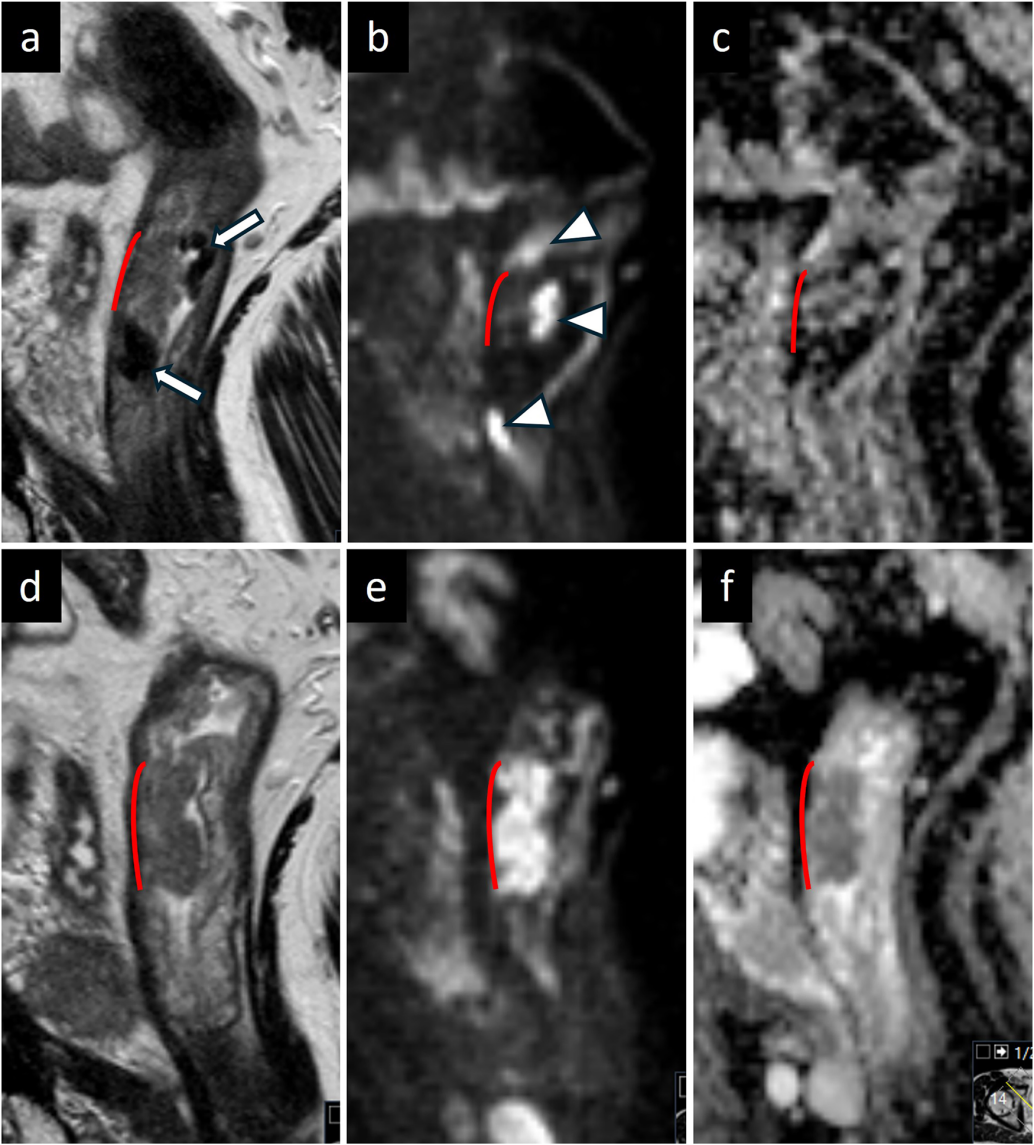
Sagittal plane MRI of a 3 cm long tumor at the anterior rectal wall, (a–c) without rectal micro-enema, and (d–f) with micro-enema. a T2 TSE, it is difficult to delineate the oral border of the tumor, and the tumor is partly surrounded by feces and gas. b DWI b-1000, difficult to identify the tumor, which is surrounded by hot spots. c ADC, difficult to identify the tumor. After the micro-enema, the tumor is well displayed on (d) T2 TSE, (e) DWI b-1000, and (f) ADC. Red line, tumor base; white arrow, feces or gas; white arrow heads, hot spots
